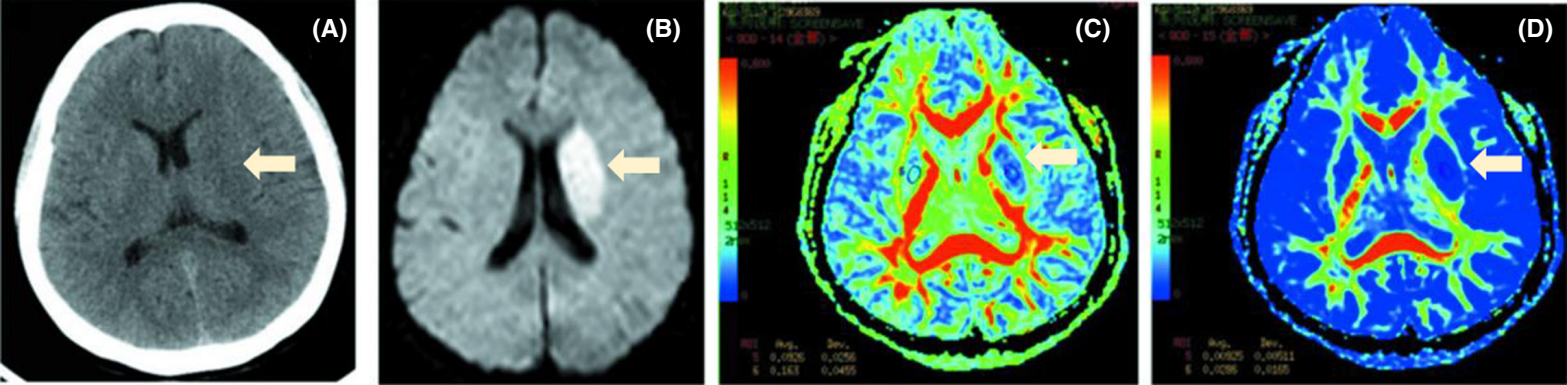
(A) Computed tomography of the brain. (B) Brain magnetic resonance imaging showed an acute ischemic stroke in the left basal ganglia region. (C, D) Diffusion tensor imaging
